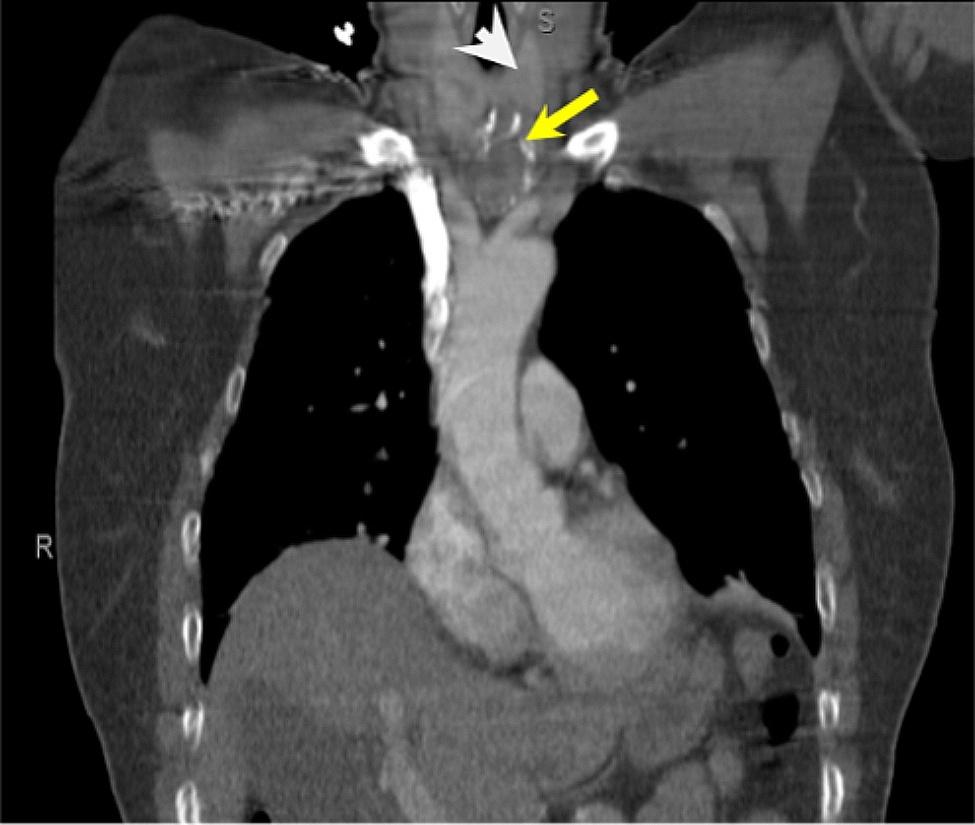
Chest CT-scan shows a heterogenous mass (yellow arrow) with peripheral calcifications measuring 40 × 33 × 28 mm, inferior to the left thyroid lobe (white arrowhead) and extending into the upper mediastinum
